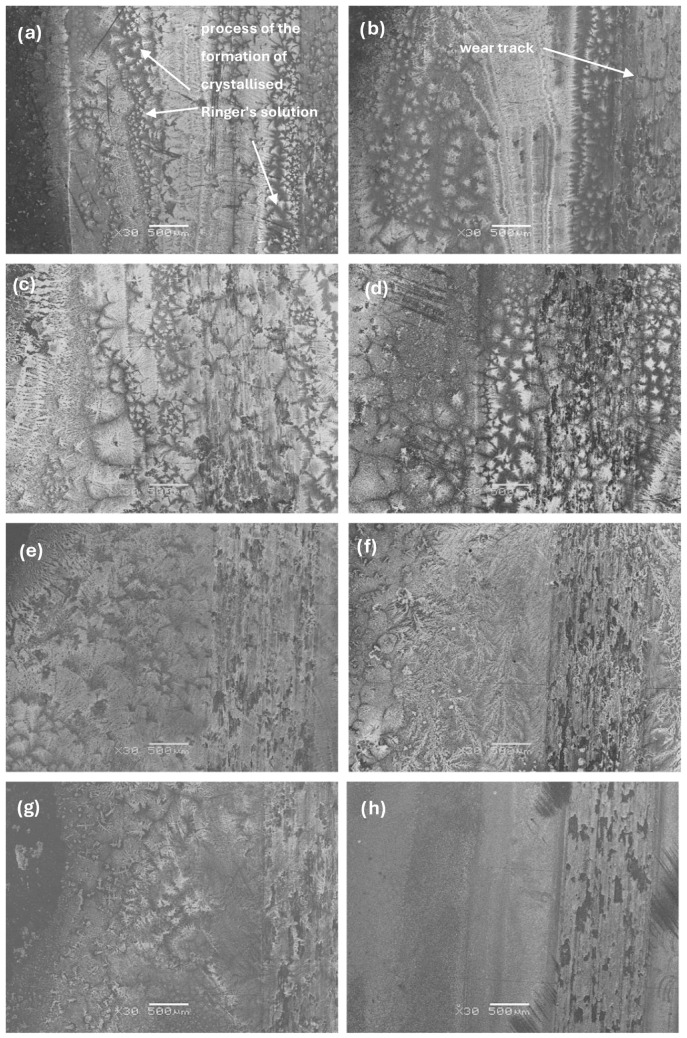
SEM images of wear tracks before ultrasound cleaning for samples: (a) Zr-10Nb, (b) Zr-10Nb-2Mo, (c) Zr-10Nb-4Mo, (d) Zr-10Nb-6Mo, (e) Zr-10Nb-8Mo, (f) Zr-10Nb-10Mo, (g) Zr-10Nb-12Mo, and (h) Zr-10Nb-14Mo.
